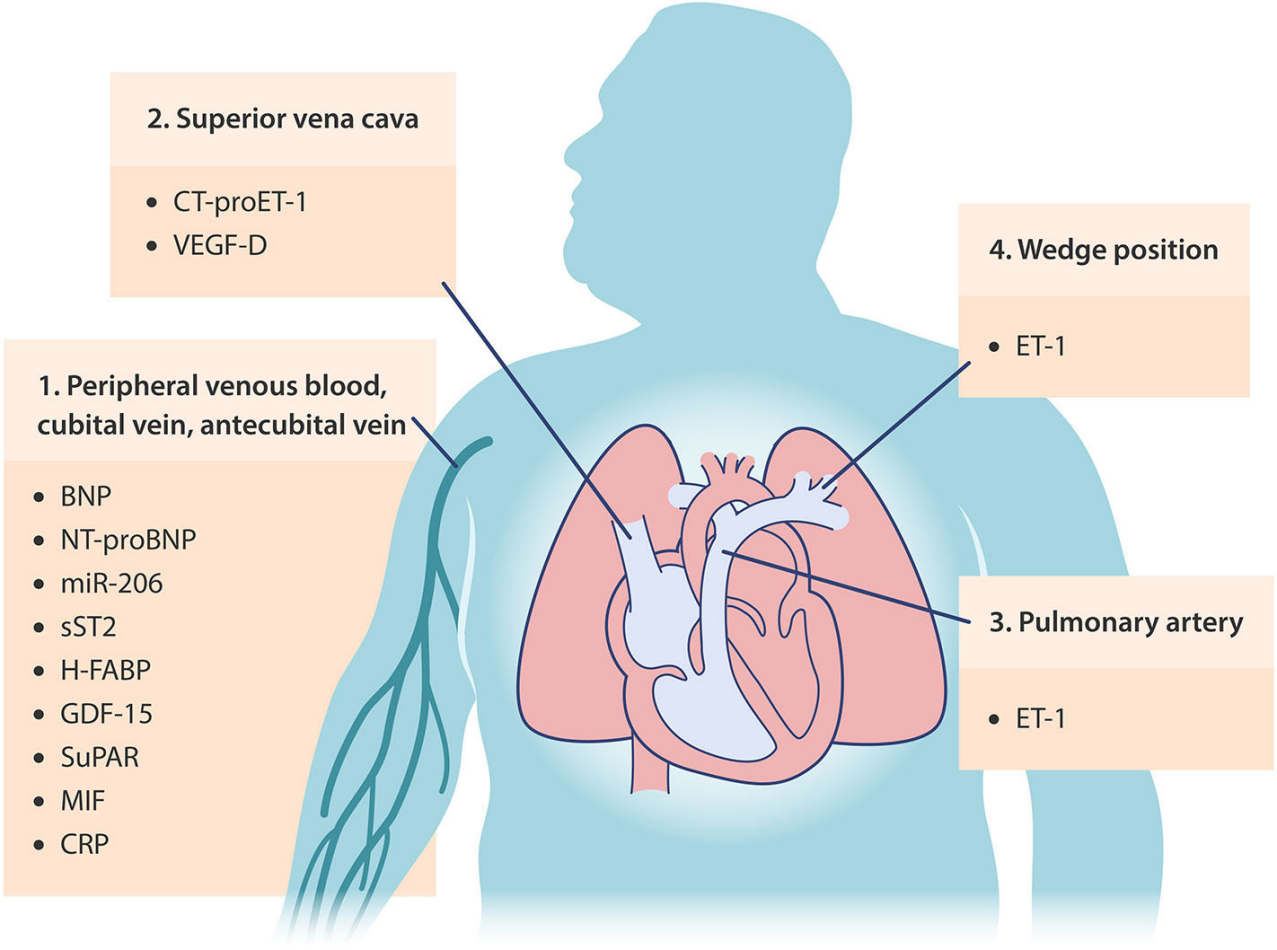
Occurrence locations of potential circulating biomarkers in PH-LHD. BNP, brain/B-type natriuretic peptide; NT-proBNP, N-terminal proBNP; miR-206, microRNA-206; sST2, soluble suppression of tumorigenicity 2; H-FABP, heart type fatty acid binding protein; GDF-15, growth differentiation factor 15; suPAR, soluble urokinase plasminogen activator receptor; MIF, macrophage migration inhibitory factor; CRP, C-reactive protein; ET-1, endothelin-1; CT-proET-1, C-terminal pro-ET-1; VEGF-D, vascular endothelial growth factor-D.
